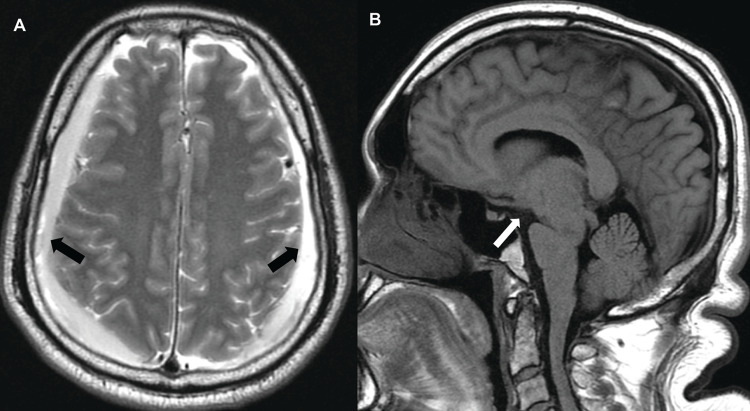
Initial consultation, non-contrast magnetic resonance imaging of the brain. (A) T2 axial plane demonstrating bilateral subdural hematomas (black arrows). (B) T1 sagittal plane demonstrating flattened pontomesencephalic angle (white arrow).
